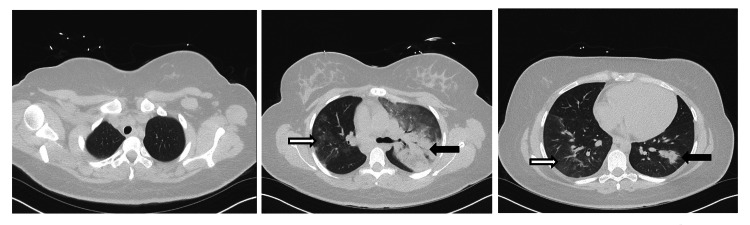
CT chest on presentation showing patchy, multilobar pulmonary consolidation and ground-glass opacities Left: upper lung zones, middle: mid lung zones at the level of left main bronchus, right: lower lung zones. Black arrows are pointing to the pulmonary consolidations, white arrows are pointing to the ground glass opacities.
